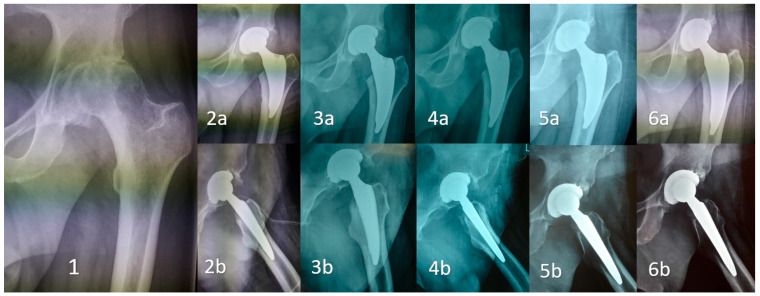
Radiographic evaluation throughout the study. (1) Preoperative anteroposterior (AP) view. (a) AP view, (b) lateral view. (2) postoperative period, (3) One-month follow-up, (4) 3-month follow-up, (5) 1-year follow-up and (6) 3-year follow-up.
